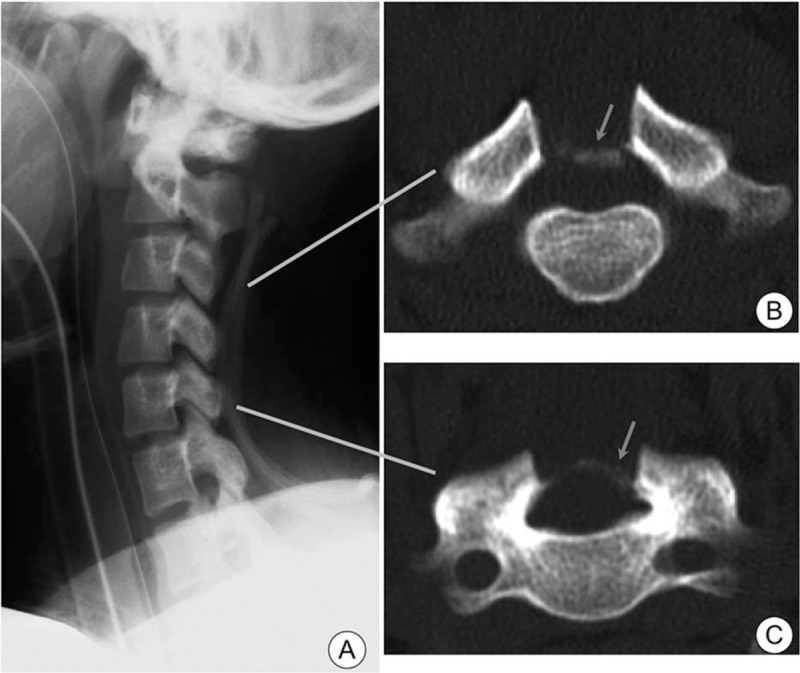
Postoperative images. C1 to C7 laminectomy was confirmed on the lateral radiograph (A). Calcification of the posterior part of the dura mater after laminectomy was observed on axial computed tomography images; representative slices at the level of C3 (B) and C5 (C) are shown.
